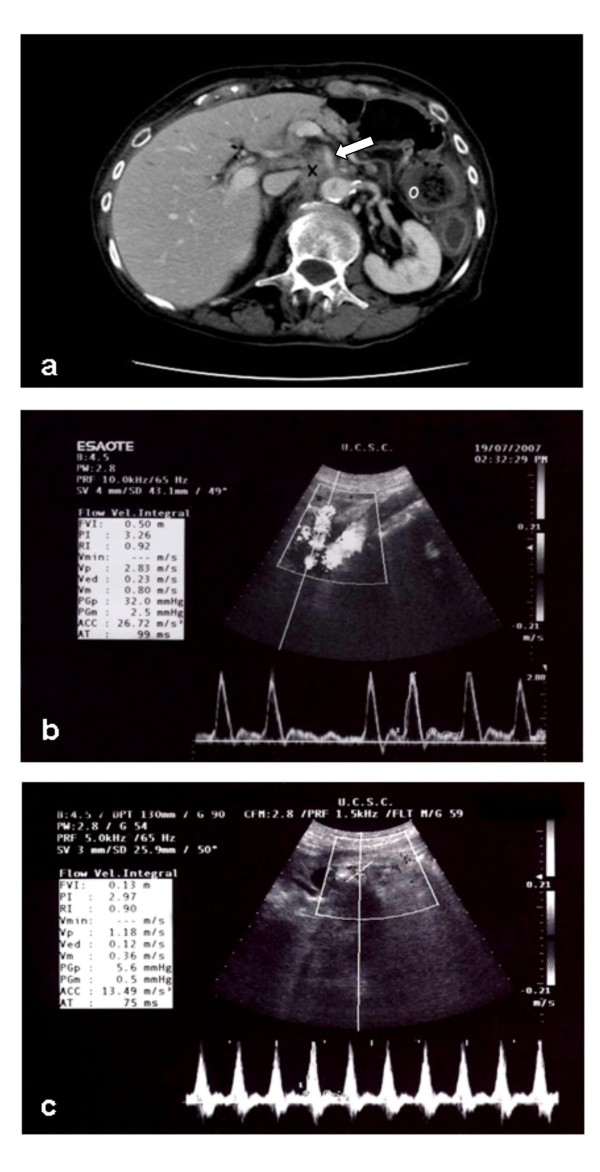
(A) Contrast-enhanced abdominal CT scan. Axial image of the origin of the superior mesenteric artery (↑) from the abdominal aorta. A hypodense mass (X) envelops the origin of the artery. Bowel loop thickening (O) is also evident. (B) Duplex ultrasound assessment of flow through the superior mesenteric artery. (C) Duplex ultrasound assessment of flow through the celiac trunk.
